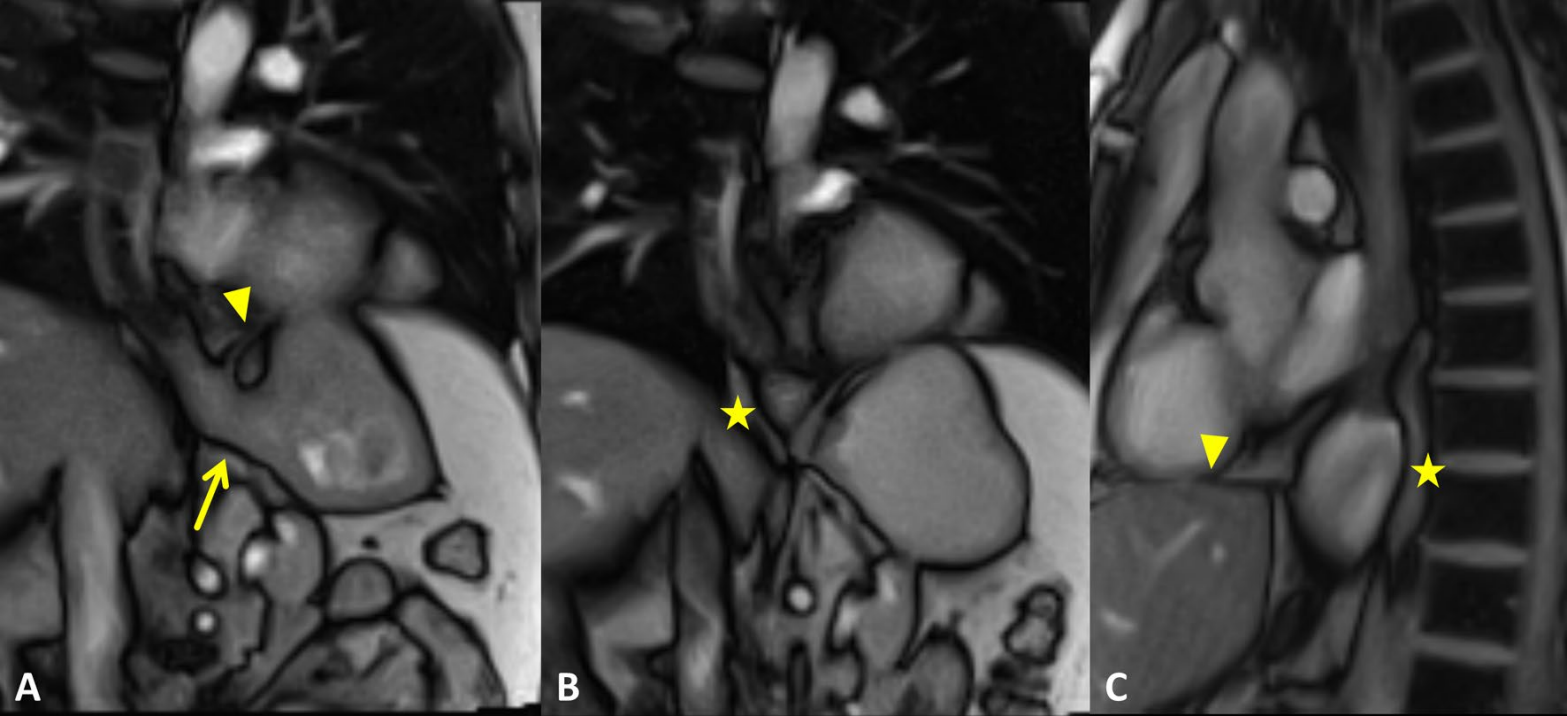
Real-time MRI of the EGJ at rest (A) and during Valsalva (B and C) in a patient with EGJ type I on HRM. MRI performed in coronal oblique planes (A and B) at sagittal planes (C). At rest, the EGJ (arrow) is clearly positioned below the diaphragm (arrowhead) (A), confirming the EGJ morphology on HRM. However, the EGJ moved above the diaphragm during Valsalva maneuver (B) and formed a small hiatal hernia (large arrow). Repetitive Valsalva maneuver resulted in a relevant size increase of the hiatal hernia (star; C). See also supplementary material Video 1
